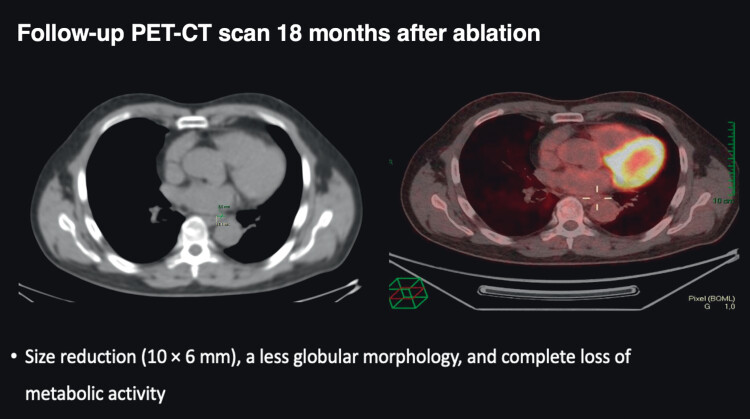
A representative image showing PET-CT imaging 18 months after
